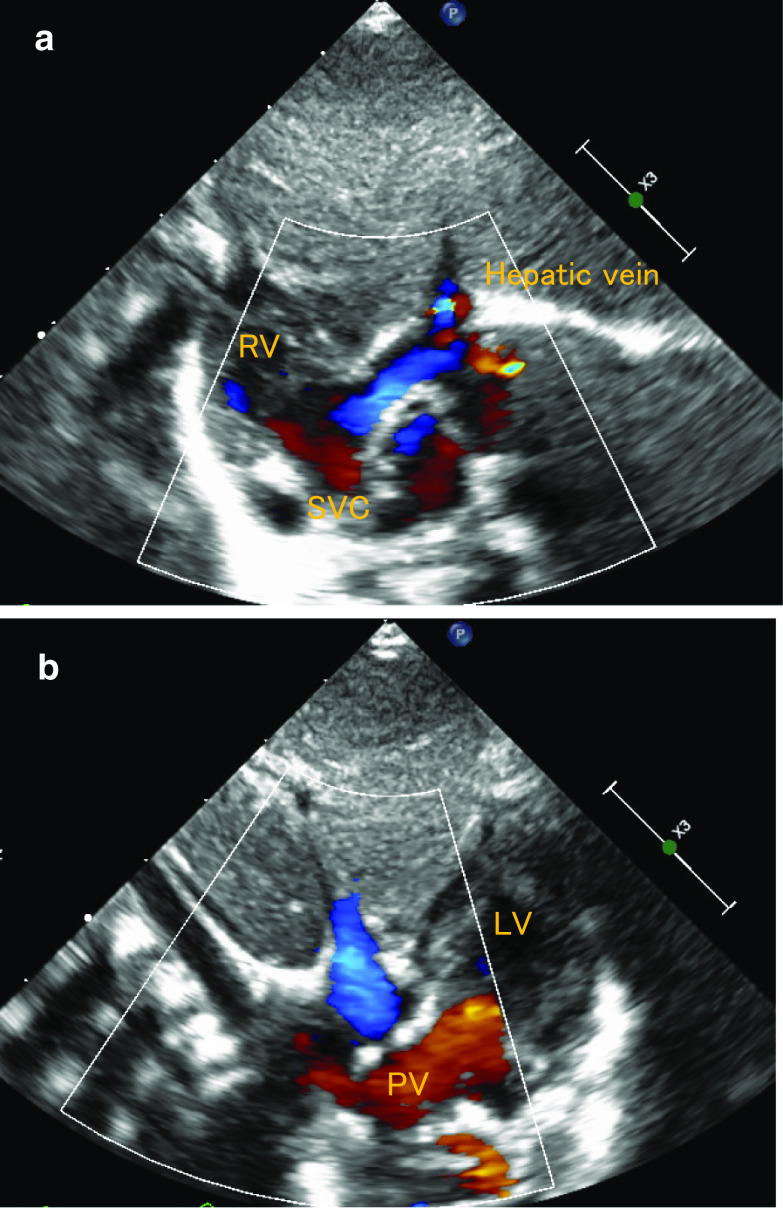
a, b The transthoracic echocardiography taken after Mustard operation showed that the systemic venous flow routed to the right-sided atrium through the systemic venous baffle, whereas the pulmonary venous flow was routed to l-A through the pulmonary venous baffle
